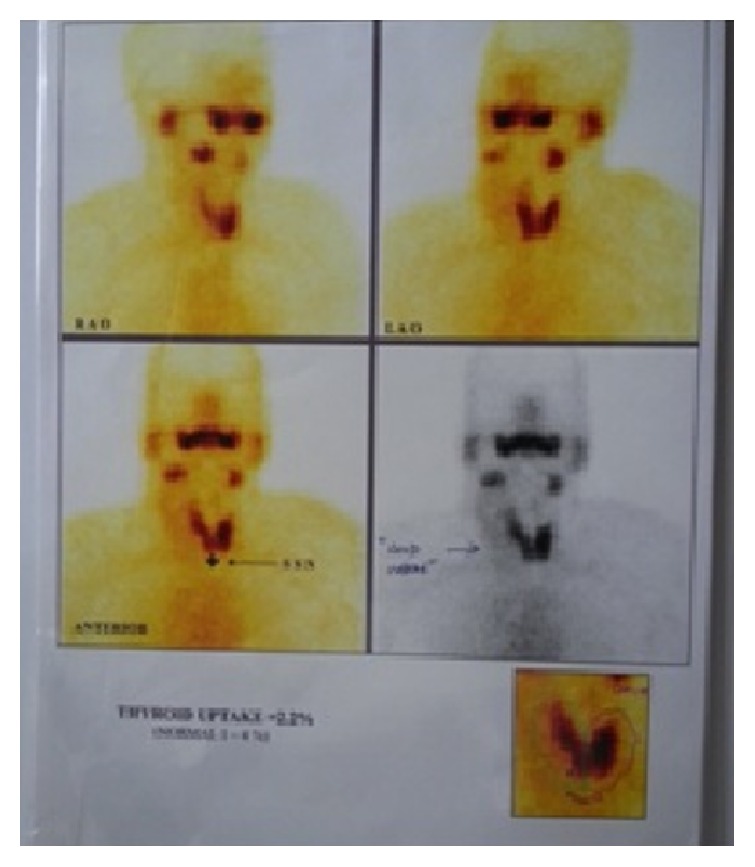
Tc99m scintigraphy showing normal uptake in the thyroid gland, a cold nodule in the right lobe, and normal activity in the salivary gland.
